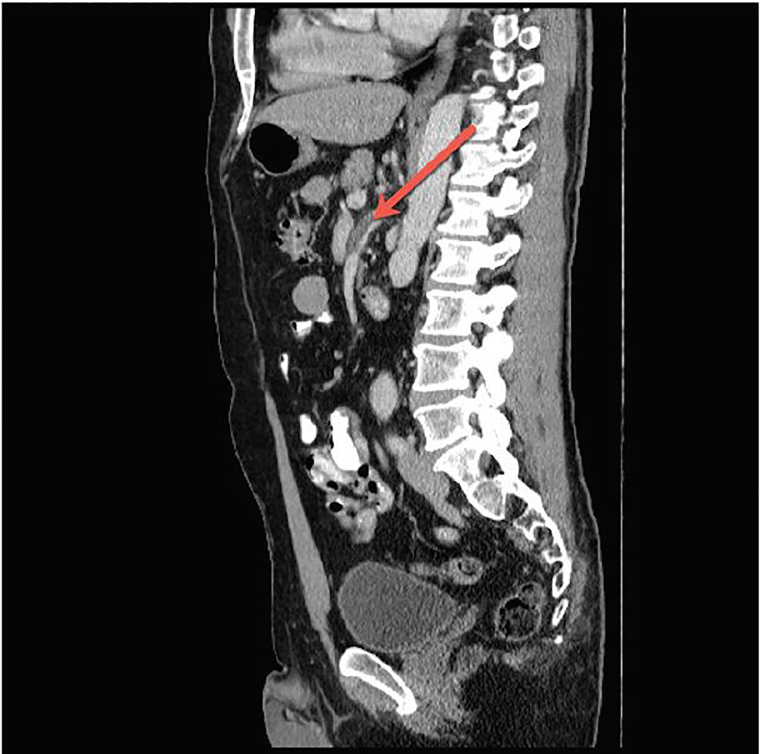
Sagittal computed tomography with intravenous contrast demonstrating thrombus (arrow) in the false lumen of the superior mesenteric artery dissection.
